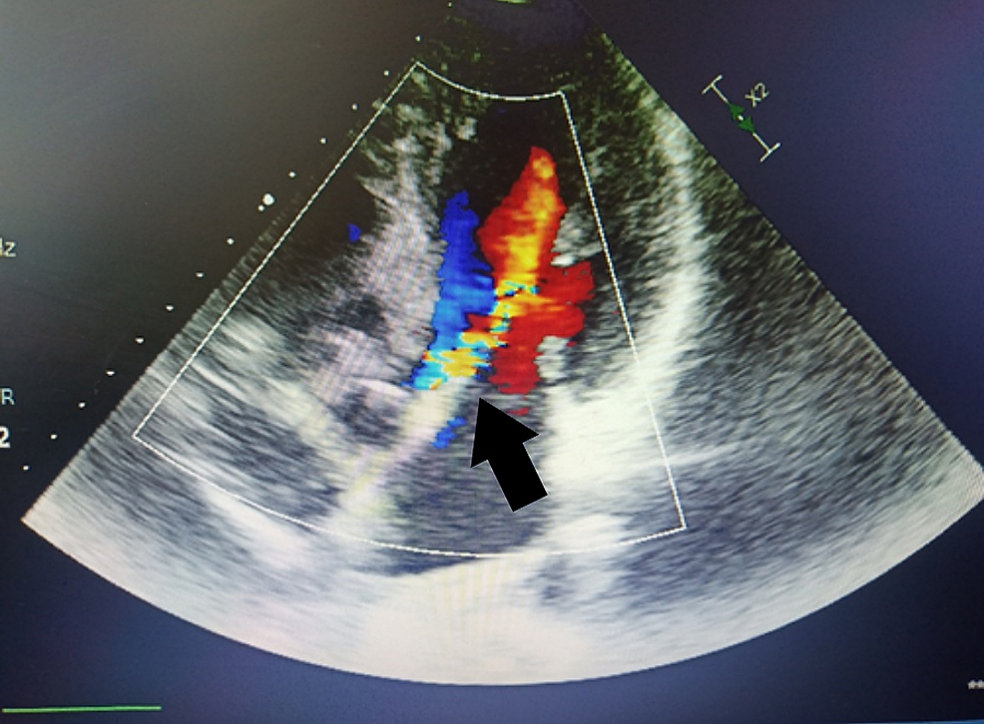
Transthoracic echocardiography. The color Doppler five-chamber view shows the finding of aortic valve insufficiency.
